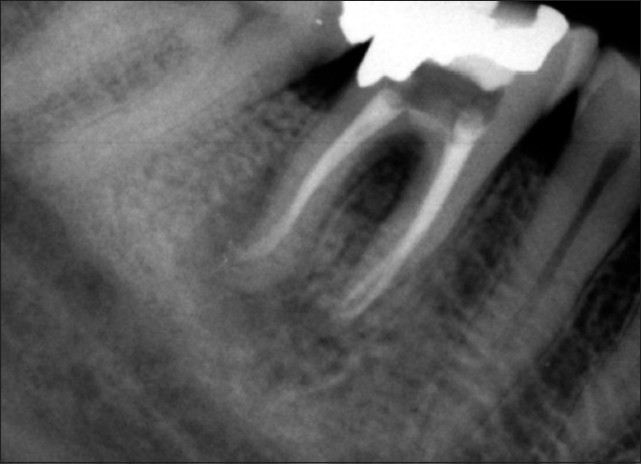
Periapical image after treatment
